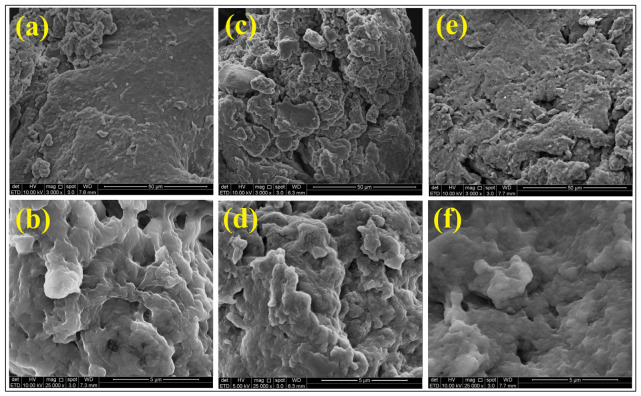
SEM of (a,b) CB, (c,d) CT, and (e,f) CS beads at 3000× magnification and 25,000× magnification after the adsorption of ER.
